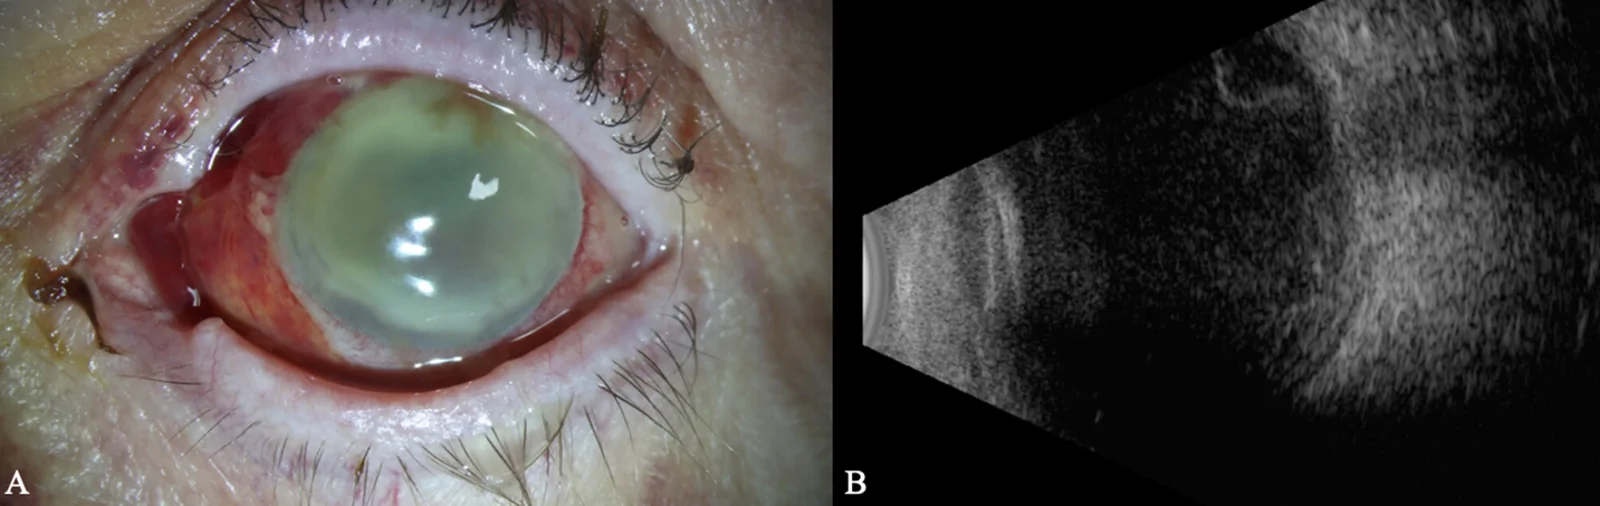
Patient 1 left eye. (A) Slit lamp photography showing diffuse injection, corneal ring infiltrate, and hypopyon. (B) B-scan ultrasound showing vitreous opacities and early membrane formation. Despite treatment, best corrected visual acuity at last follow-up was no-light-perception and the eye underwent enucleation
